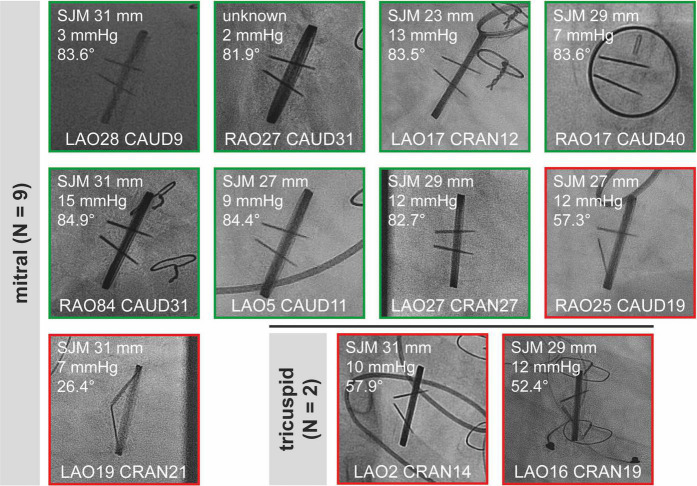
Sufficient cinefluoroscopy studies of MHVs in mitral and tricuspid position. MHVs had either normal leaflet opening and closing (green) or leaflet dysfunction (red). MHVs are shown during maximum opening. Individual images show the opening angle (for details refer to Figure 1), mean gradient from transthoracic echocardiography, and projection angles in CF. For comparison, the mean opening angles of SJM, ATS, and Carbomedics MHVs are 83.4, 69.5, and 77.1°, respectively (12). Some images are already shown in Figure 1. CAUD, caudal; CRAN, cranial; LAO, left anterior oblique; MHV, mechanical heart valve; RAO, right anterior oblique; SJM, St. Jude Medical.
